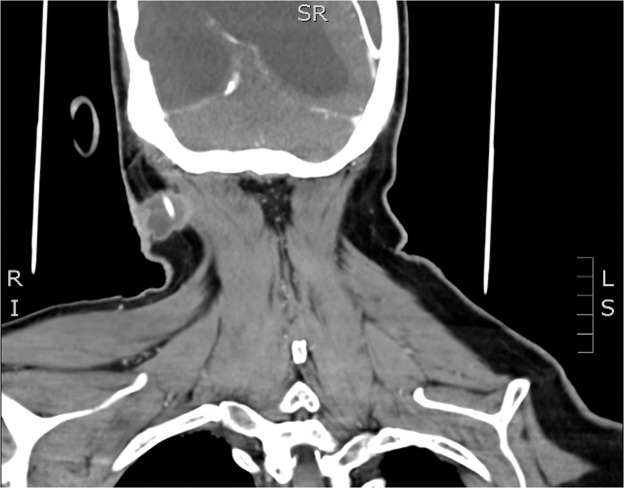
Pre-operative CT demonstrating location of cutaneous lesion encasing shunt catheter, adhering to skeletal muscles.
